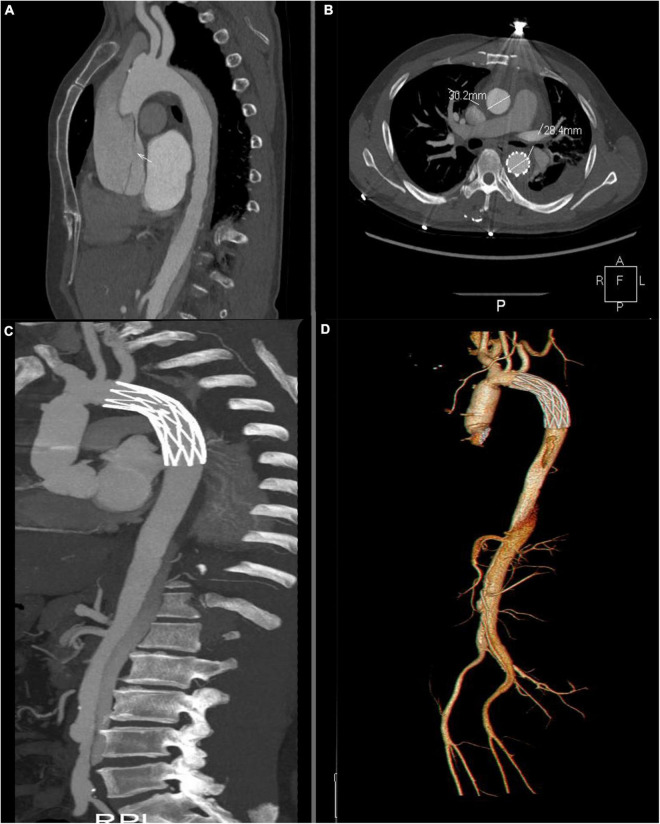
Computed tomography angiography assessment. (A) Intimal tear in the ascending aortic before the operation. The white arrow indicates the intimal tear. (B) Postoperative examination at 12 month. Thrombosis of the thoracic false lumen in descending aortic. (C,D) Postoperative volume-rendered image.
